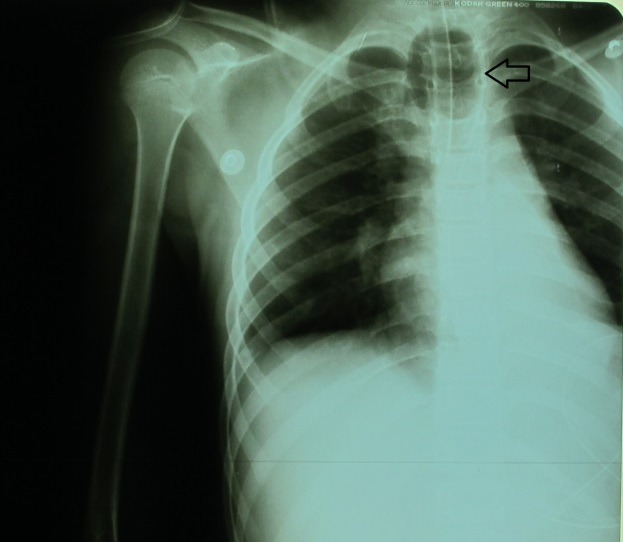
Chest x-ray showing the hyperinflated endotracheal cuff
